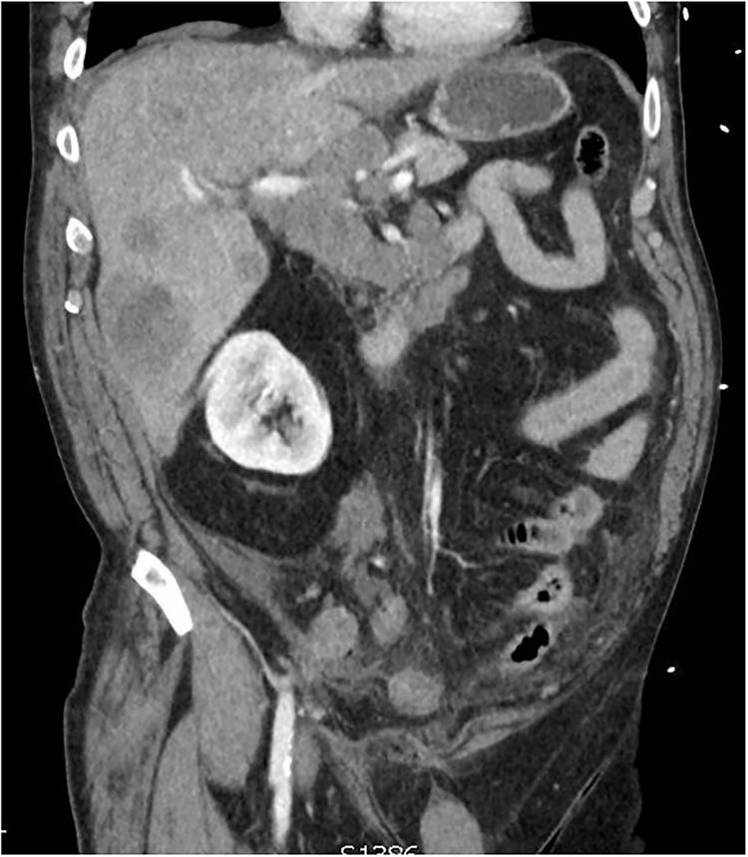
CT of the abdomen from admission demonstrating hepatic lesions, lymphadenopathy and a perforated appendix.
